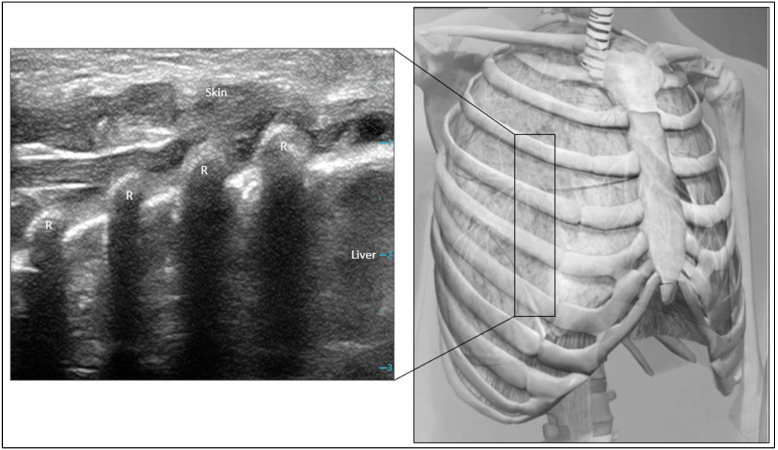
Lung ultrasound exam (left). Three intercostal spaces were scanned, anterior (NDL), lateral, and posterior (DL), on each side. R—rib. Image (right) Courtesy Visible Body.
